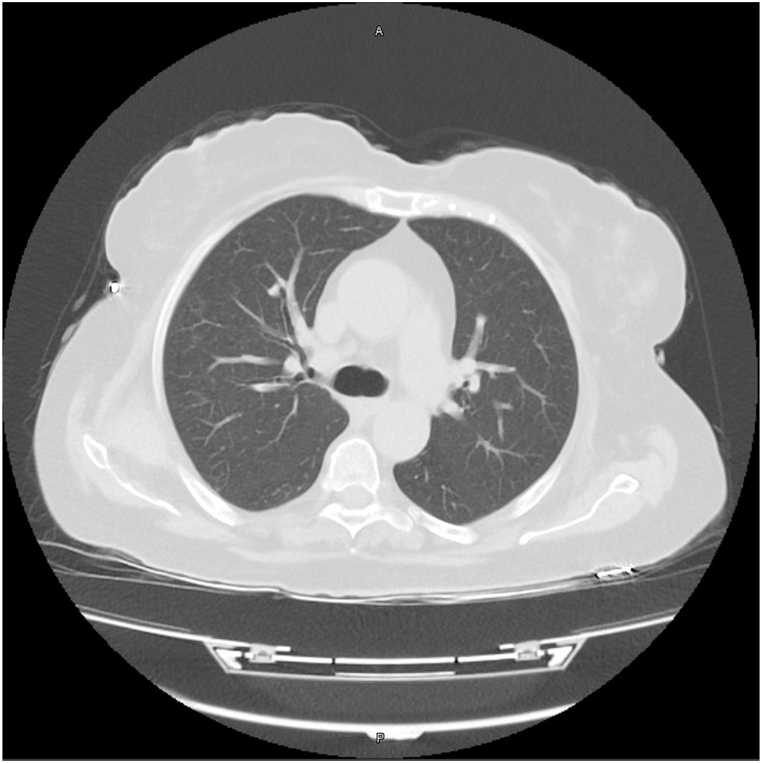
A follow-up Axial CT scan after a month of Mesalazine discontinuation showing complete resolution of the previously described lung lesions.
